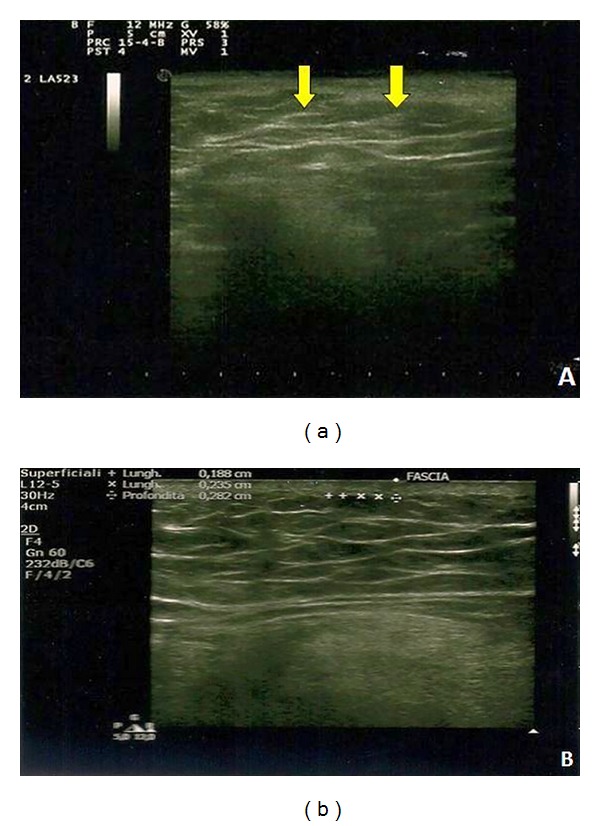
(a) Preoperative ultrasonography showed discontinuous fascia; (b) 3-month postoperative ultrasonography showed continuous fascia.
